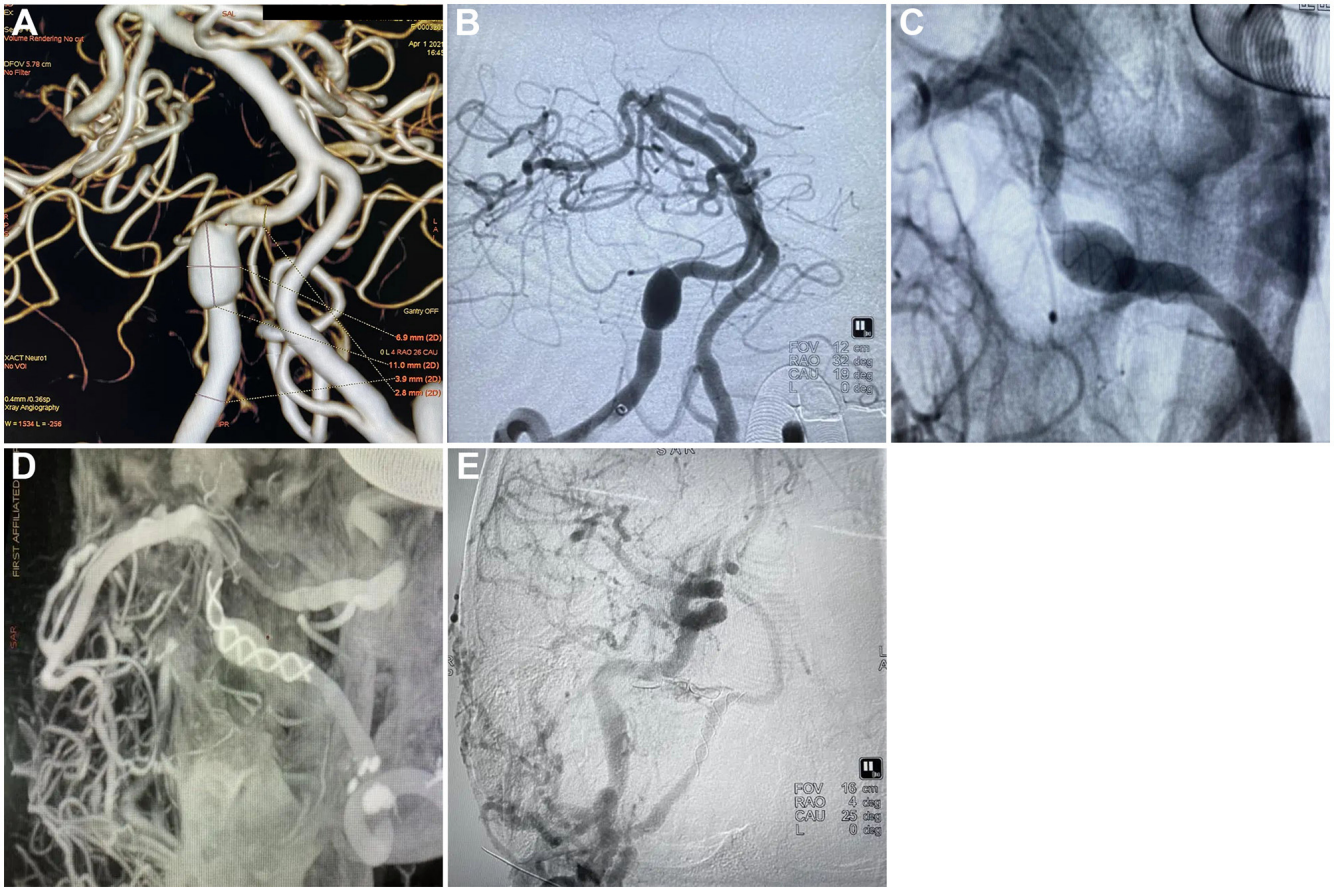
Tubridge treatment on vertebral artery dissecting aneurysm and the follow-up. (A) CTA scan showing the dissecting aneurysm in the right vertebral artery, section 4 (V4); (B) DSA showing the dissecting aneurysm in the right vertebral artery, section 4 (V4); (C) Release of Tubridge flow diverter (3.5 × 55 mm); (D) DSA showing a goof wall apposition after final deployment of the flow diverter; (E) Follow-up 6 month after the treatment found no silhouette of the dissecting aneurysm.
